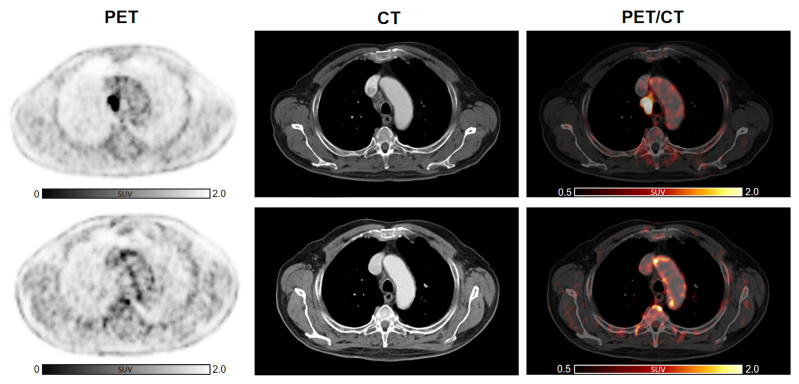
Increased 2-[^18^F]FDG arterial uptake after ICI therapy compared to baseline in a subject without any other previous anti-cancer treatment. Cancer immunotherapy with ICI resulted in a modest but significant increase in arterial 2-[^18^F]FDG in a patient not previously treated with other anti-cancer therapies, such as CHT or RT. Abbreviations: CHT, chemotherapy; 2-[^18^F]FDG, 2-[^18^F]fluoro-d-glucose; ICI, immune checkpoint inhibitors; RT, radiotherapy.
